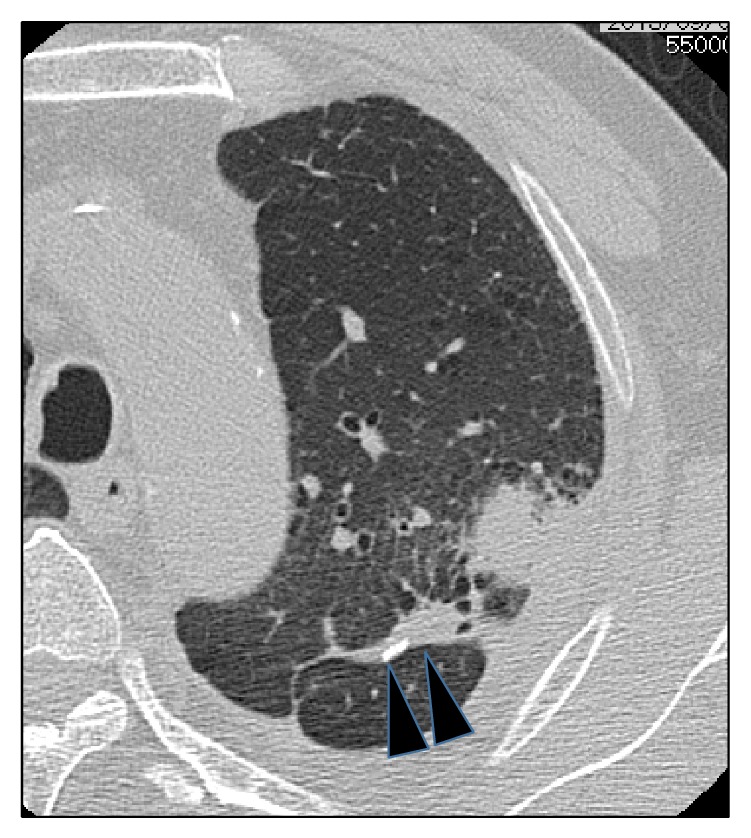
Chest CT reveals a 30 mm pulmonary nodule close to the initial surgical margin (arrowhead).
